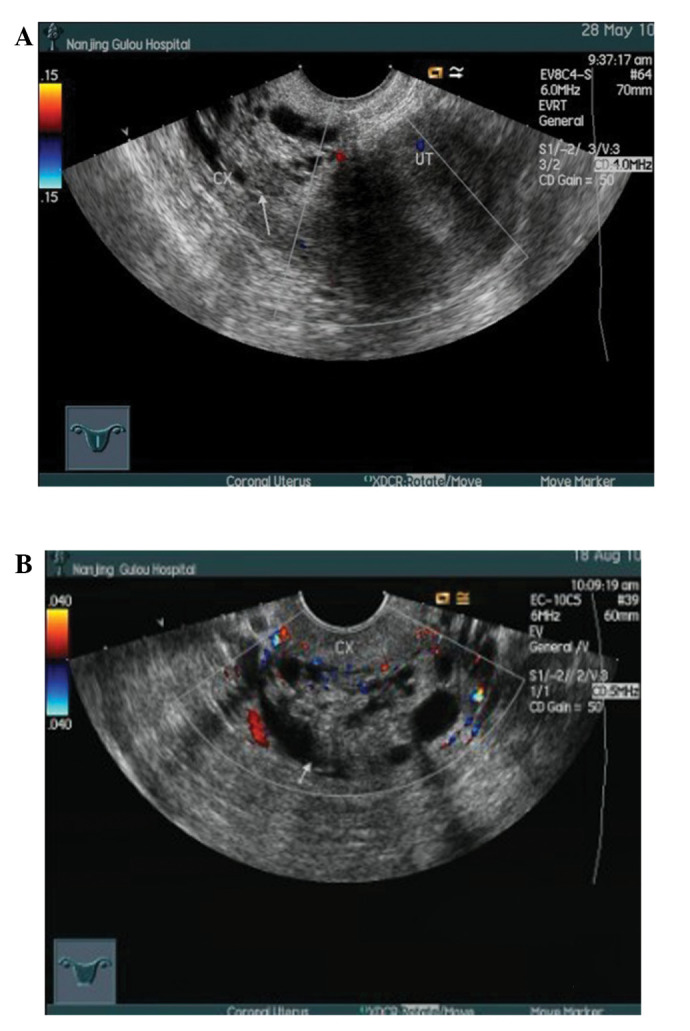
Transvaginal ultrasonographic characteristics of minimal deviation adenocarcinoma of the uterine cervix for the first case. (A) Enlarged uterus (5.3 × 6.3 × 5.1 cm), with a honeycomb appearance. (B) Detailed information of the cervix.
